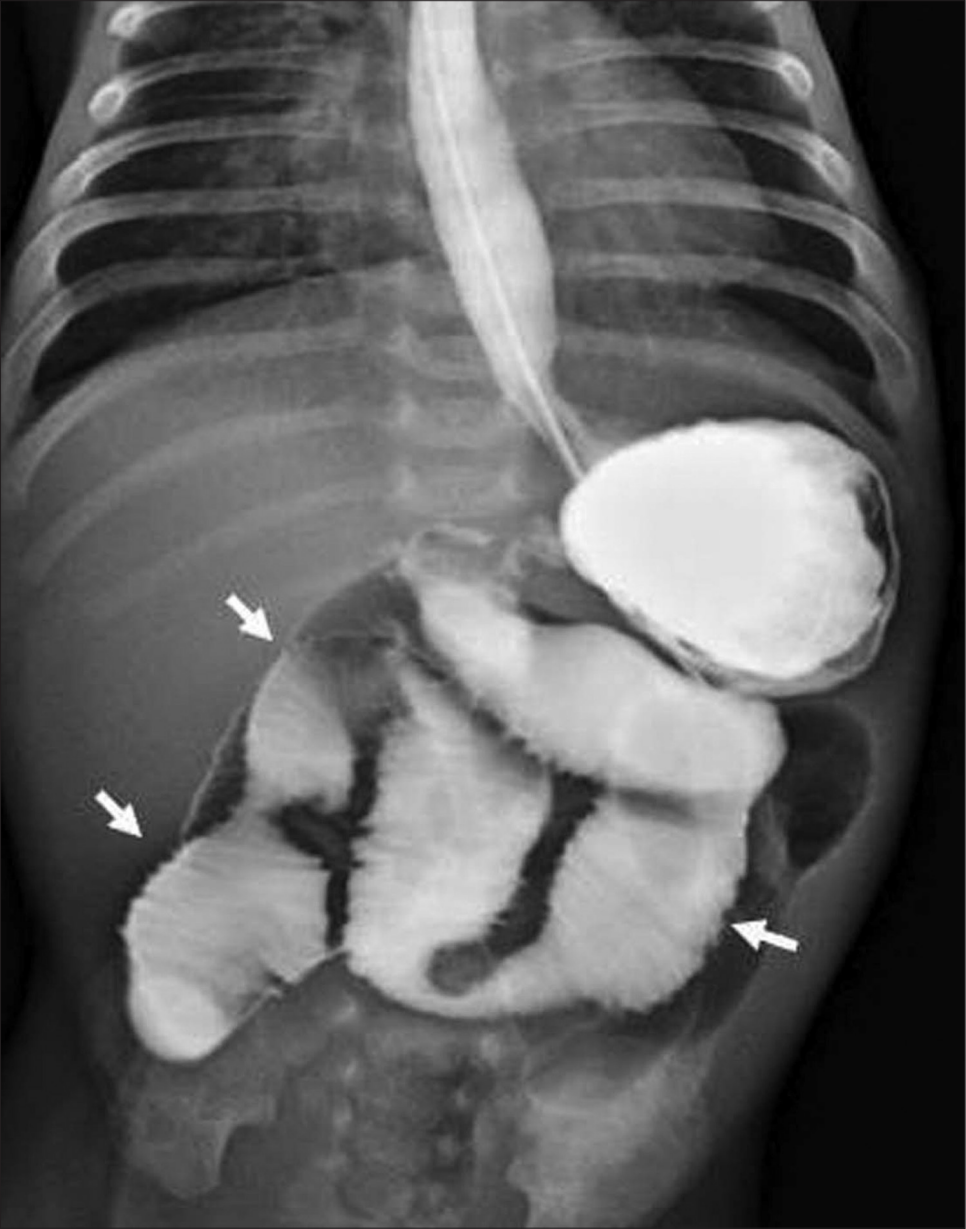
Frontal radiograph from a barium meal follow-through study shows dilated contrast-opacified small bowel (arrows); gastroesophageal reflux is seen
